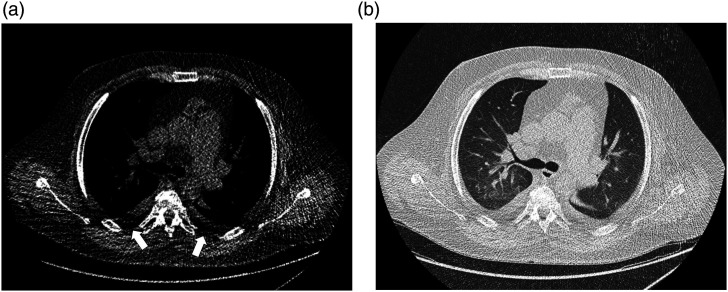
Male patient, 47 years of age, who presented with dyspnea and fever. (a) Chest CT shows minor paravertebral artifacts in surrounding soft tissues (white arrows) resulting in a quality rating of 1 (good quality). (b) Corresponding height in lung window shows no significant artifacts in lung parenchyma. DLP: 40.8 mGy*cm.
